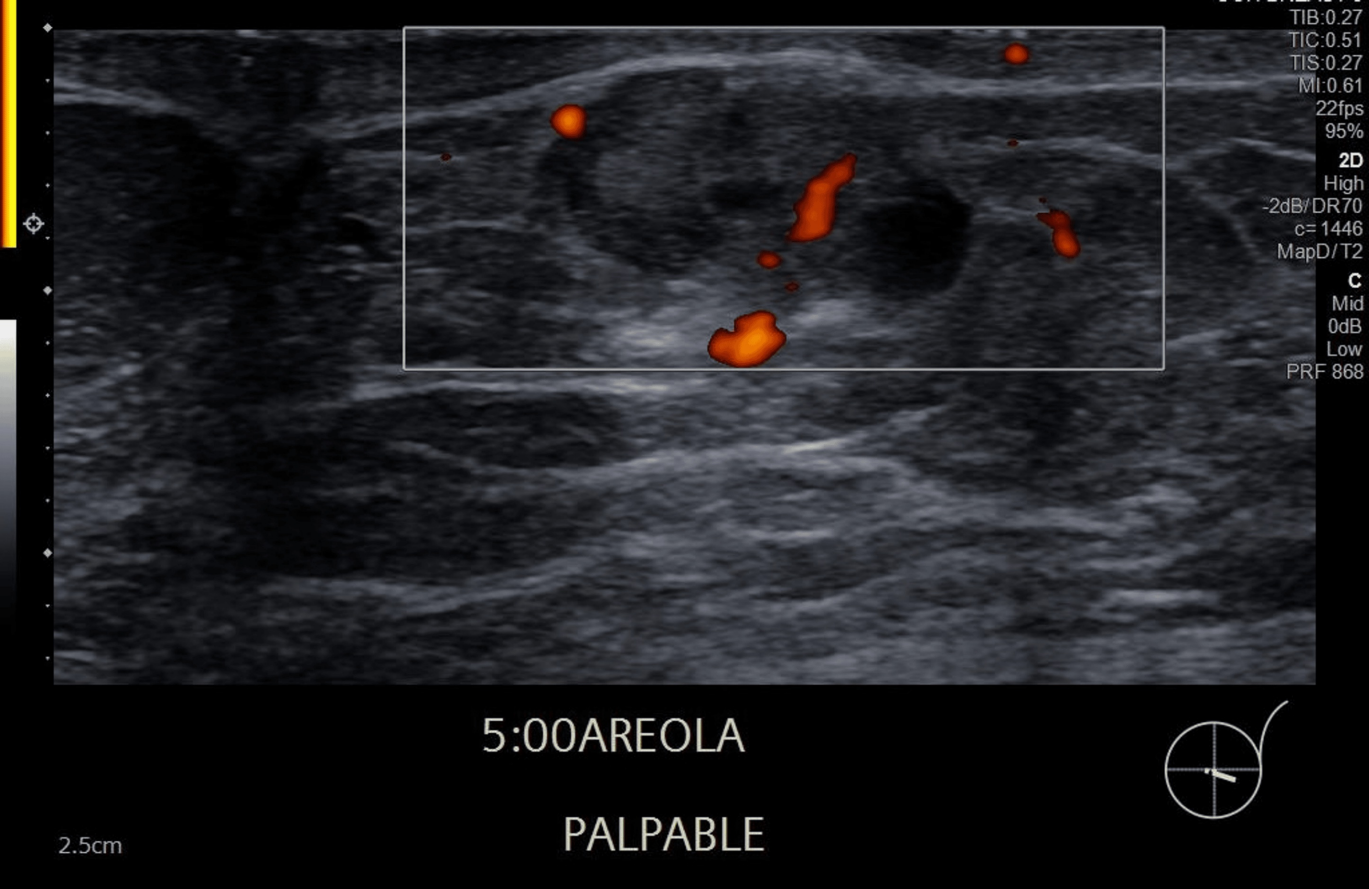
Intralesional vascularity Ultrasound revealed a lobulated, hypoechoic, heterogeneous nodule with small cystic areas and intralesional vascularity, located at the 5 o’clock subareolar region of the left breast, measuring 2.2 cm. Ultrasound-guided core biopsy confirmed invasive mucinous carcinoma (ER/PR positive, HER2 negative).
